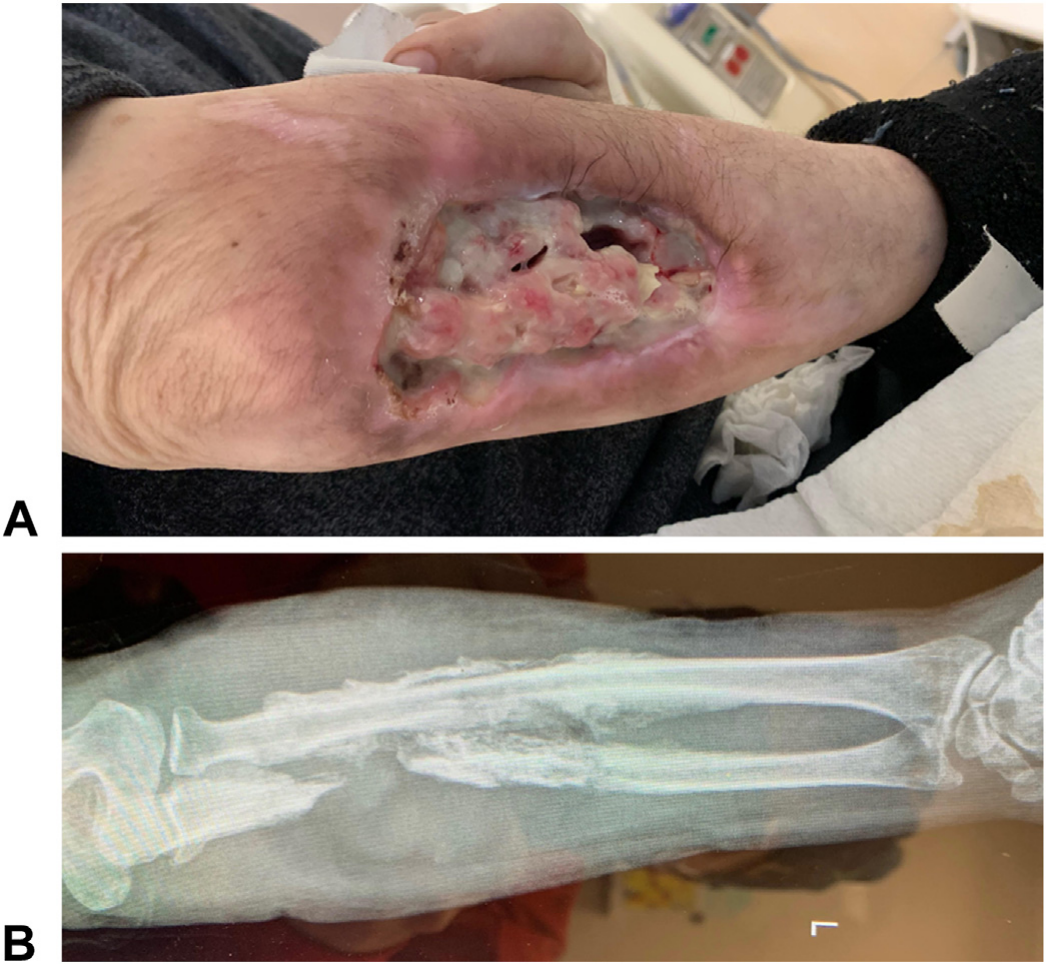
A Chronic xylazine-associated wound of the forearm with exposed ulna and secondary osteomyelitis. B Shows the X-Ray of the forearm.
